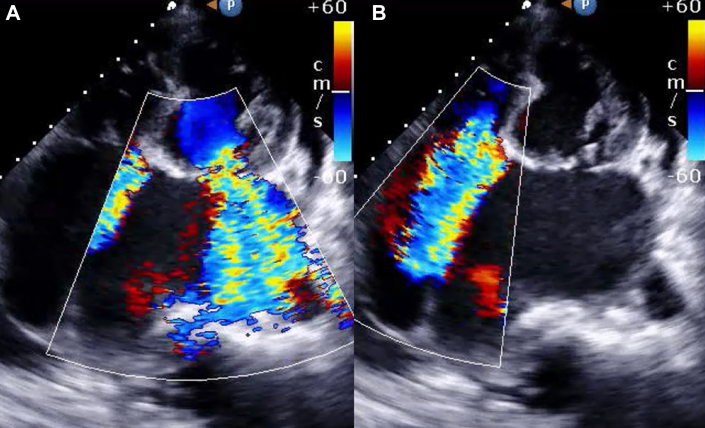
Transthoracic Echocardiography Upon Admission Apical 4-chamber view with color Doppler. (A) Severe atrial functional mitral regurgitation with a significant eccentric jet. (B) Moderate-to-severe functional tricuspid regurgitation. The massive biatrial enlargement and advanced remodeling serve as the mechanical substrate for subsequent hemodynamic deterioration.
